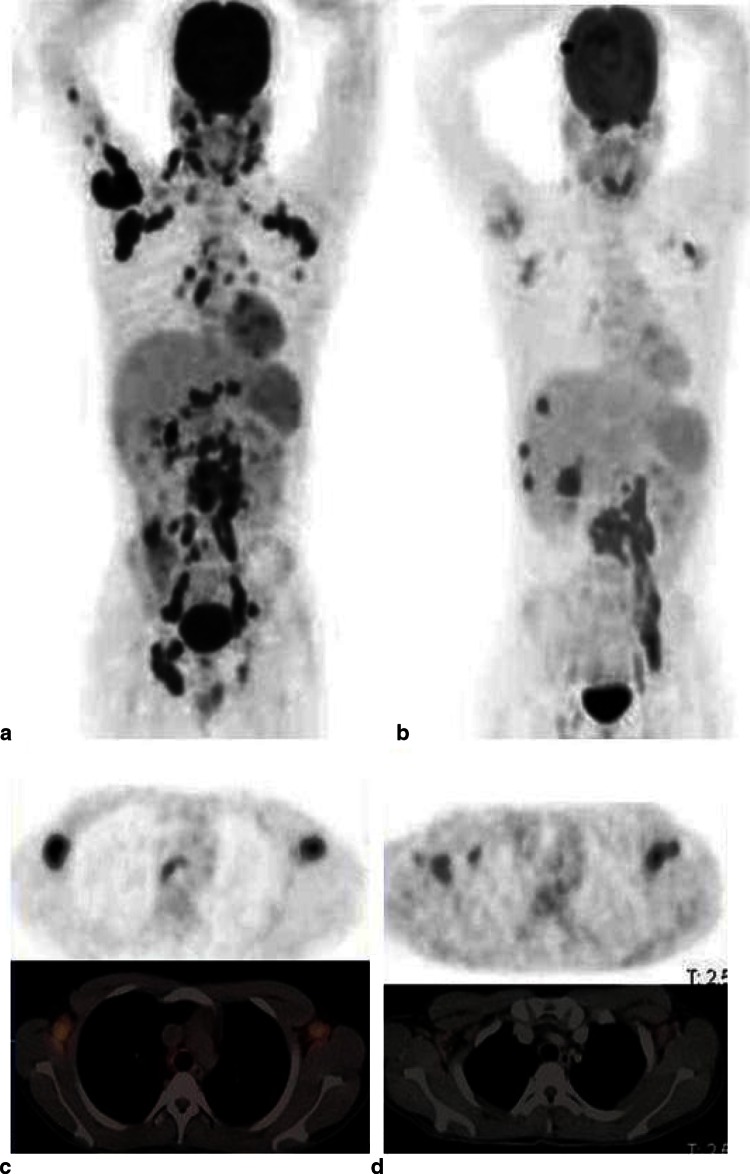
^18^F-FDG PET/CT scan before anti-TB treatment and 2 months after initiation of treatment for interim assessment of treatment response. a Maximum intensity projection (MIP) image before treatment (PET images only), showing extensive disease: pulmonary, cervical, axillary, mediastinal, abdominal, pelvic and inguinal lymph nodes, hepatic and skeletal metastasis to the lumbar spine and right humerus. b MIP image after 2 months of anti-TB treatment (PET images only): complete metabolic response of the pulmonary and right humeral lesions and the pelvic and inguinal lymph nodes. Good metabolic response in the mediastinal, cervical and axillary nodes. Active disease is still present in the lumber spine with progression of the hepatic lesions. c Transverse scans showing axillary nodes before treatment (PET and integrated PET/CT images). d Transverse scans showing response of axillary nodes after 2 month of anti-TB therapy; nodal uptake diminished but still present
